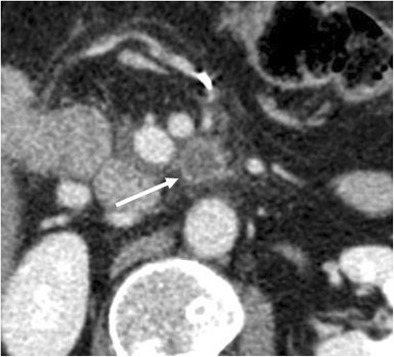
Nodal tumour recurrence. Axial CT image shows the presence of an enlarged necrotic lymph node (white arrow) along the superior mesenteric vessels, consistent with tumour recurrence
